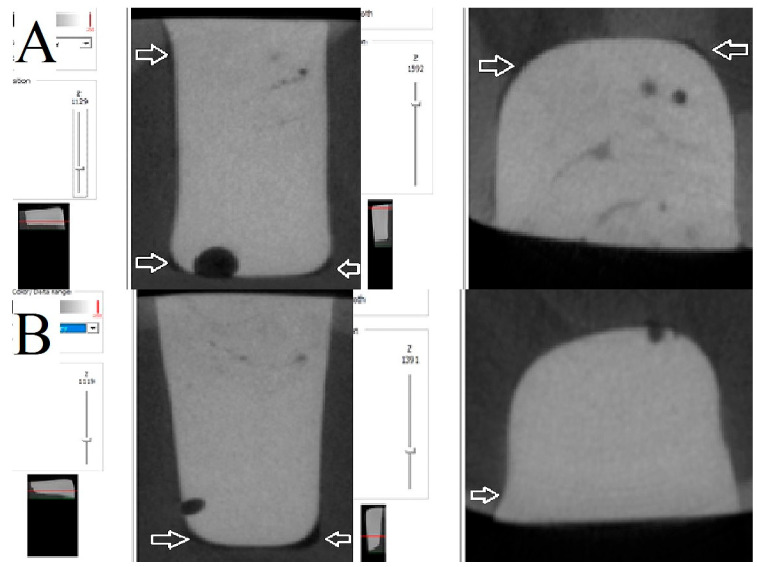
Representative images of microcomputed tomography analysis of preheated VisCalor Bulk restorations bonded with self-etch (A) and total-etch (B) adhesives. The presence of white arrows denotes the location of the internal gap.
